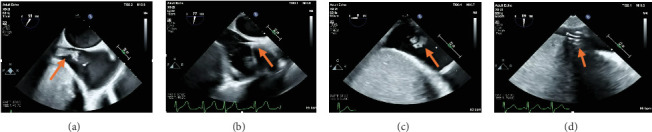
Intraprocedural transesophageal imaging displaying (a) right atrial thrombus extending from the IVC (orange arrow). (b) AngioVac debulking of IVC thrombus (orange arrow). (c) Hepatic vein thrombus (orange arrow). (d) AngioVac debulking of hepatic vein thrombus (orange arrow).
